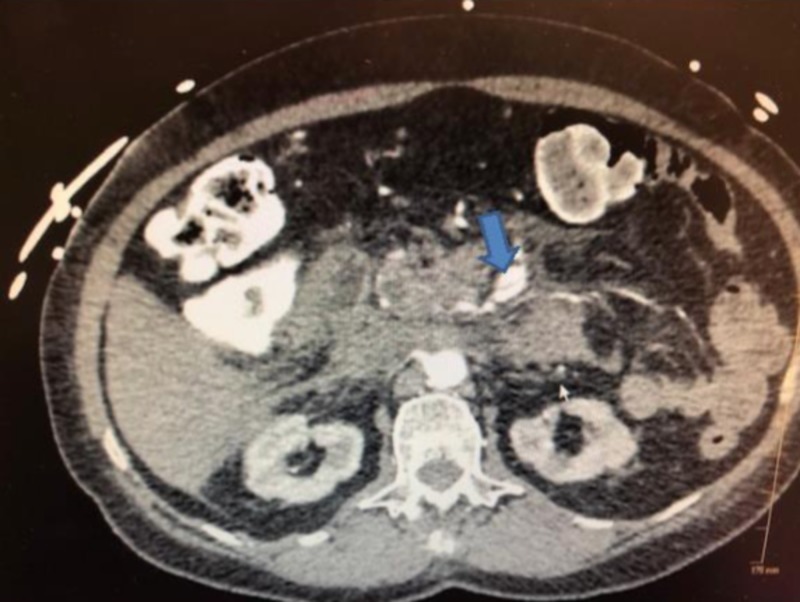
CT scan showing dissection of superior mesenteric artery and intimal flap.
